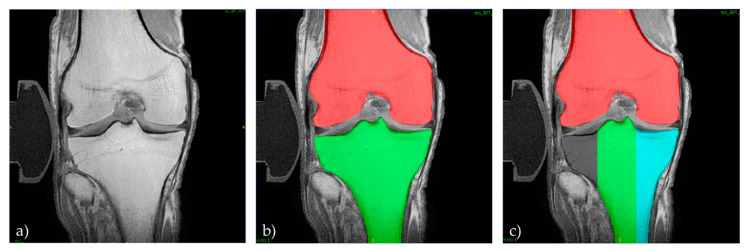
Manual segmentation procedure. For each joint, configuration, and condition, the coronal images of the T1-weighted sequence (a) were manually segmented and labelled as femur (red) and tibia (green) (b). The tibia was further compartmentalized into the medial (turquoise) and lateral (grey) tibial condyles and the intercondylar eminence was spared (c).
